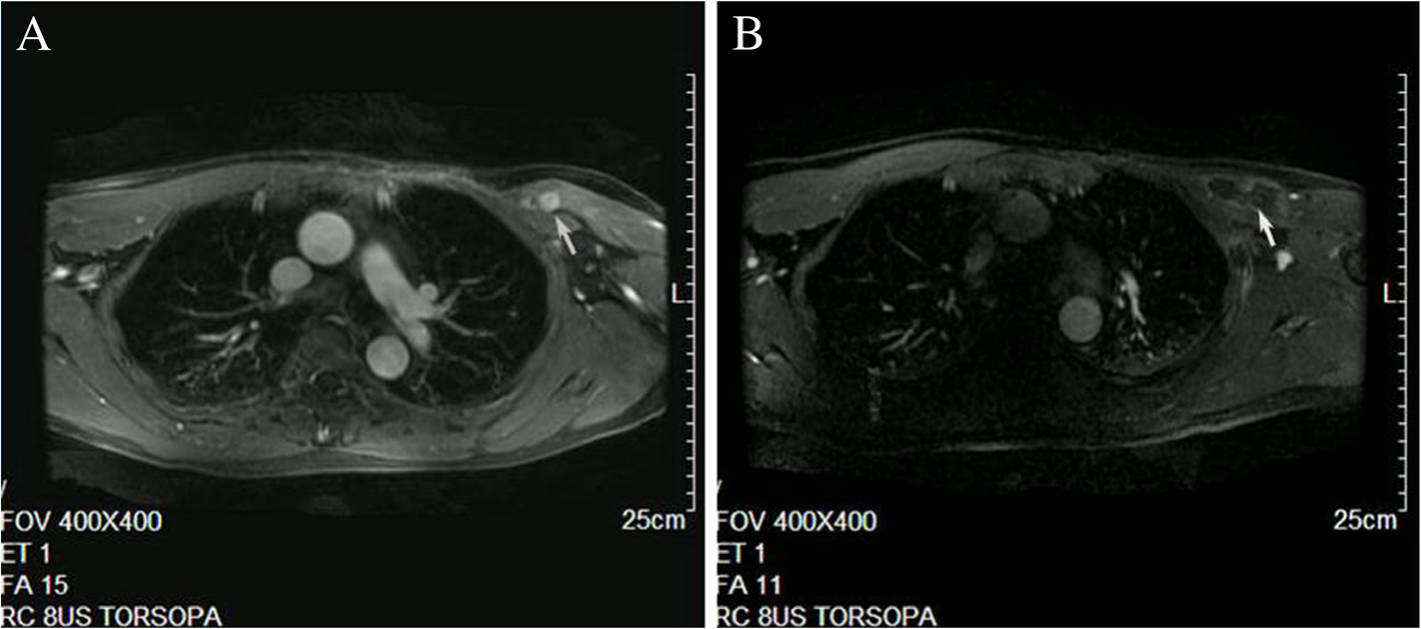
Chest MRI. (A) Before treatment, chest MRI shows enhanced tumor. (B) After treatment, arterial phase-enhanced MRI showed tumor was completely necrotized.
